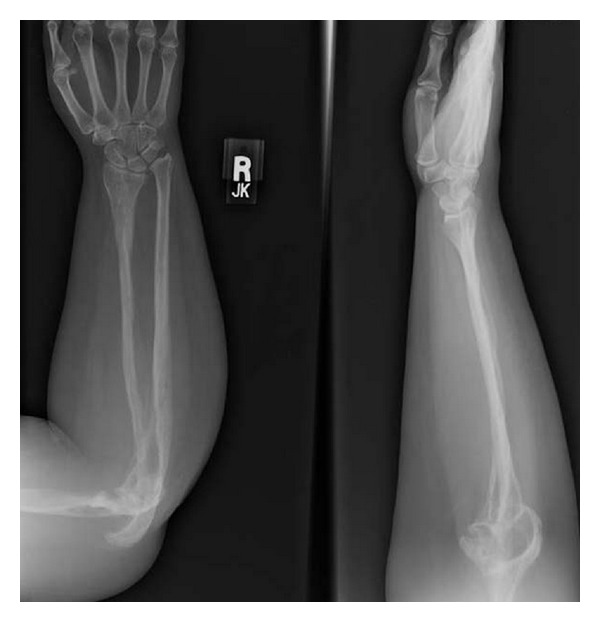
Anteroposterior and lateral plain radiographs of the forearm with multiple subcortical radiolucent foci of both the radius and ulna and subluxation of the distal radioulnar joint.
